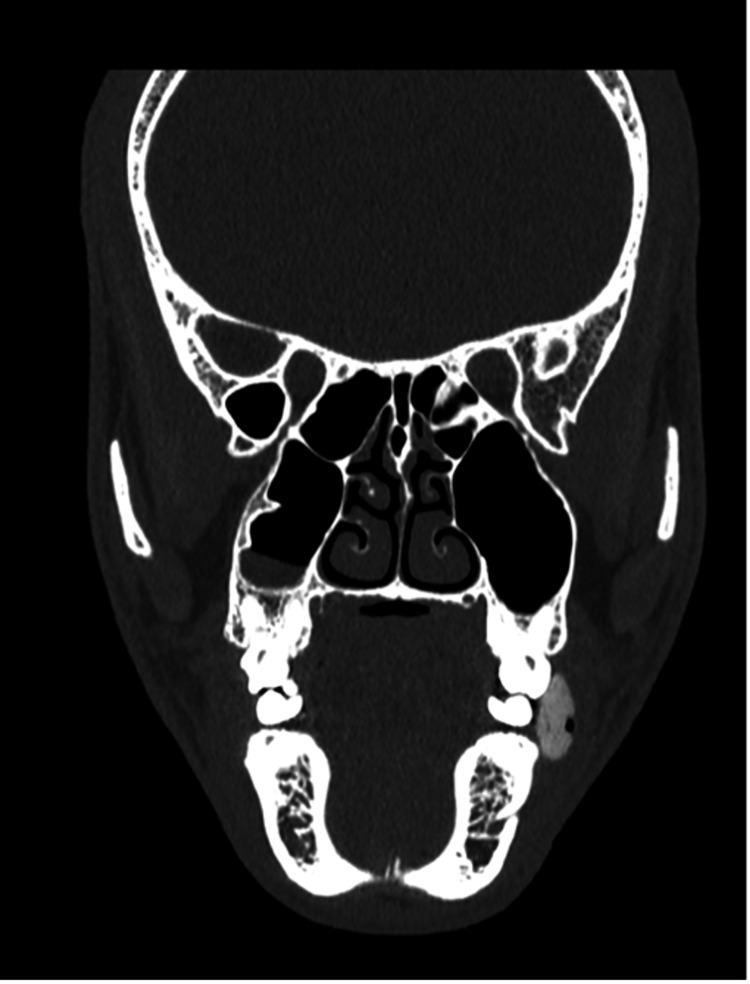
Coronal CT image in the bone window showing a circumscribed, ovoid, hyperdense mass containing a few air locules in the inferior left buccal space adjacent to the left lower molar teeth. CT: computed tomography
